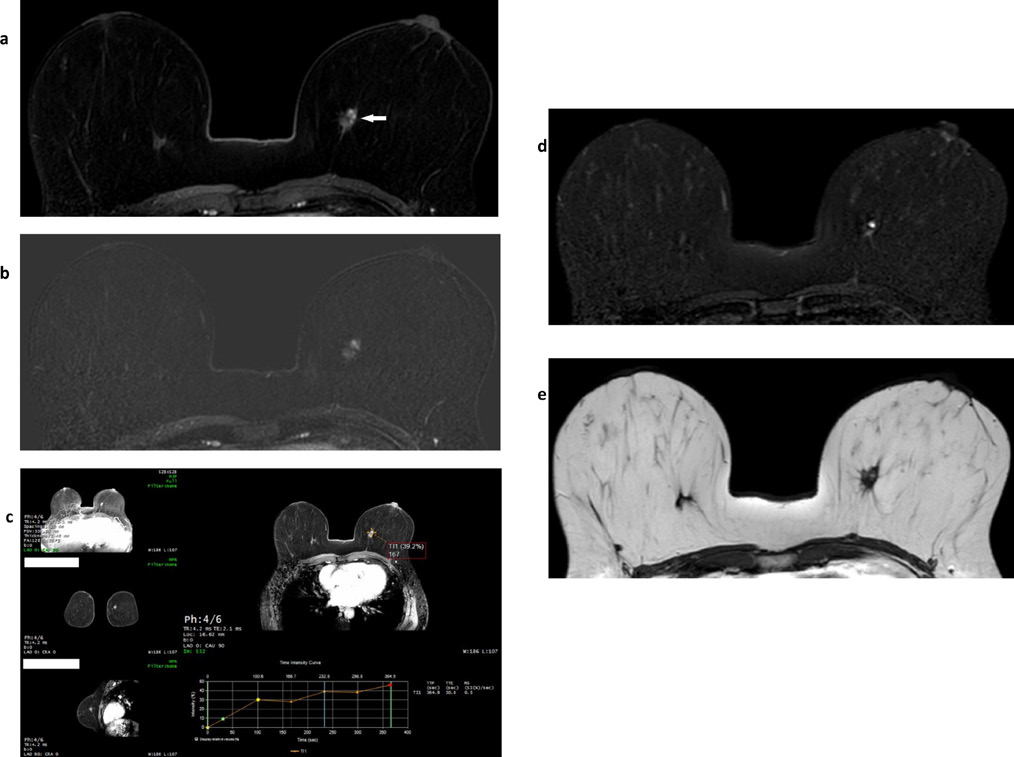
Fat necrosis. Post bilateral breast reduction, a poorly circumscribed mass (white arrow), with heterogenous internal enhancement, within the scar in the lower left breast is shown on a fat suppressed T1W image from the dynamic series (a) and on the equivalent subtracted image (b). Kinetically, it has a slowly rising persistent enhancement curve (c). It is also heterogenous with some high signal on fat suppressed T2W (d). However, on non-fat-suppressed T1W, although a partially spiculate morphology is demonstrated, there is high signal within the lesion that matches the signal intensity of the surrounding fat and enabled a diagnosis of fat necrosis within a scar to be made on MRI without biopsy (e). The symmetry of scarring from the breast reduction is also noted on this image.
